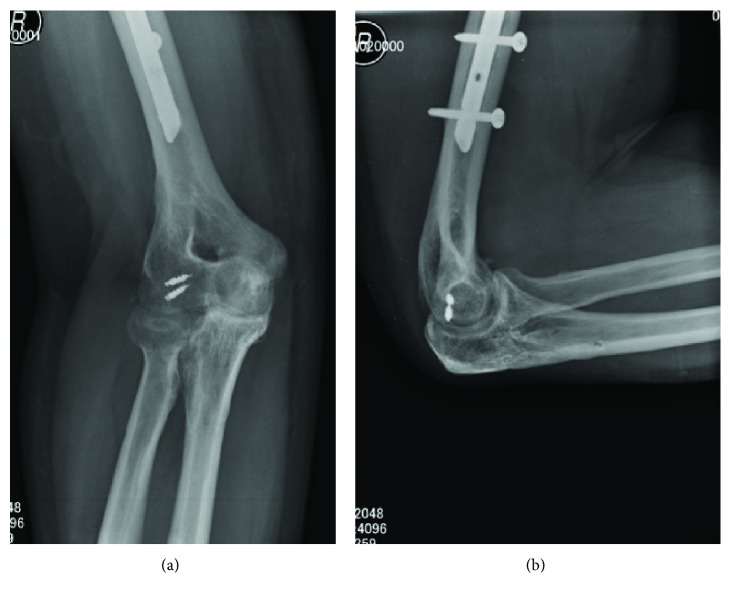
Radiographs 2 years after surgery. (a) AP view and (b) lateral view. Humeroradial joint congruency is good without osteoarthritic change.
